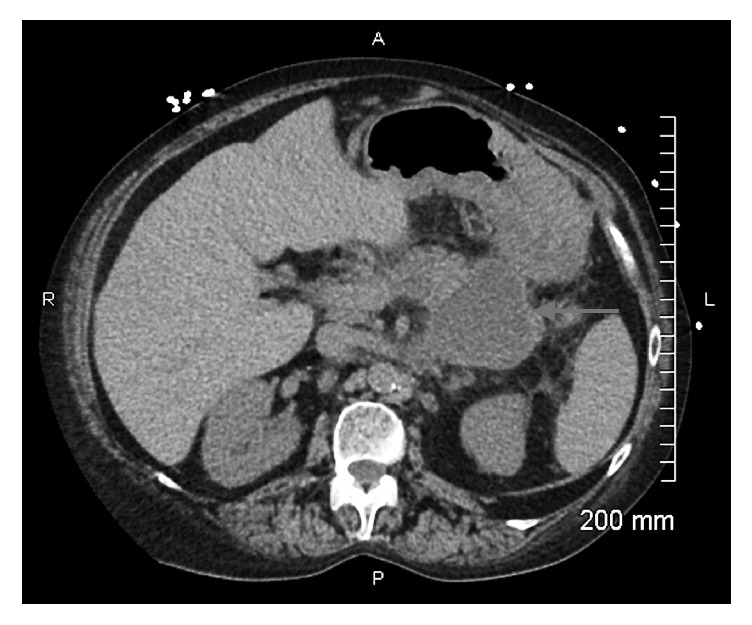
CT image; pancreatic tail lesion.
